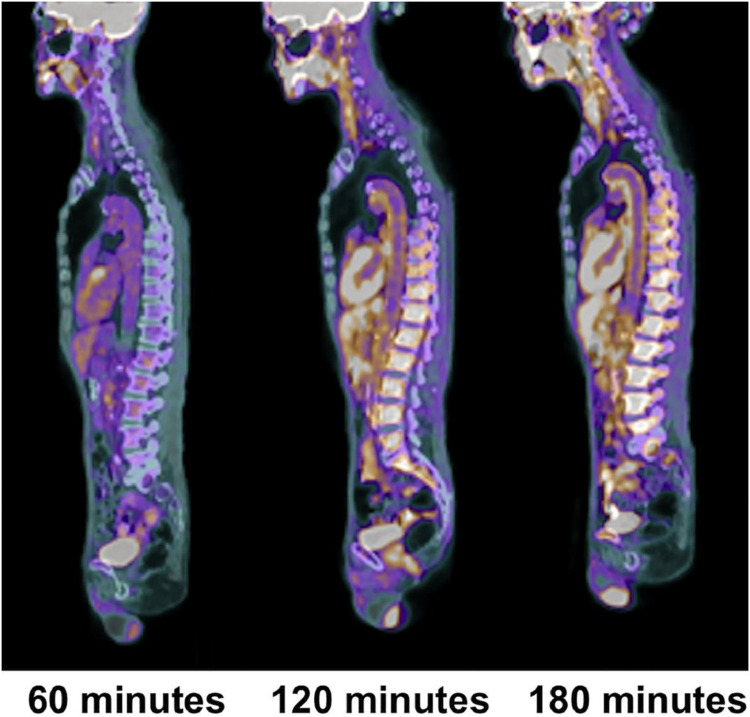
Changes in aortic wall and luminal blood FDG activity at different imaging time-points as seen on sagittal FDG PET images of the thoracic aorta. With time, luminal blood activity decreases while the aortic wall activity increases, which improves the arterial wall-to-blood contrast (superior target-to-background ratio) (Reproduced from Moghbel et al. (Gonzalez and Trigatti, 2017)with permission).
